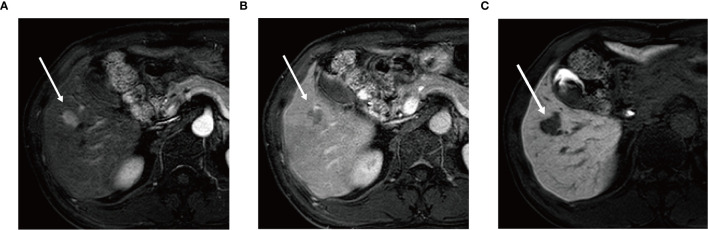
Gadoxetic acid-enhanced MR image (A) in arterial phase shows a 2.0 cm × 1.6 cm nodular lesion with arterial enhancement in hepatic segment V (arrow) and this nodule shows washout (arrow) in the portal venous phase (B). In hepatobiliary phase (C) the lesion shows wedge-shaped peritumoral hypointensity area (arrow).
